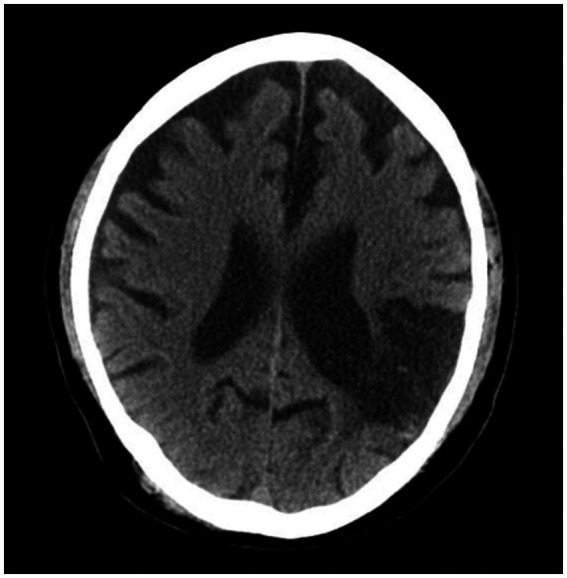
Non-contrast CT scan obtained from outside hospital.
